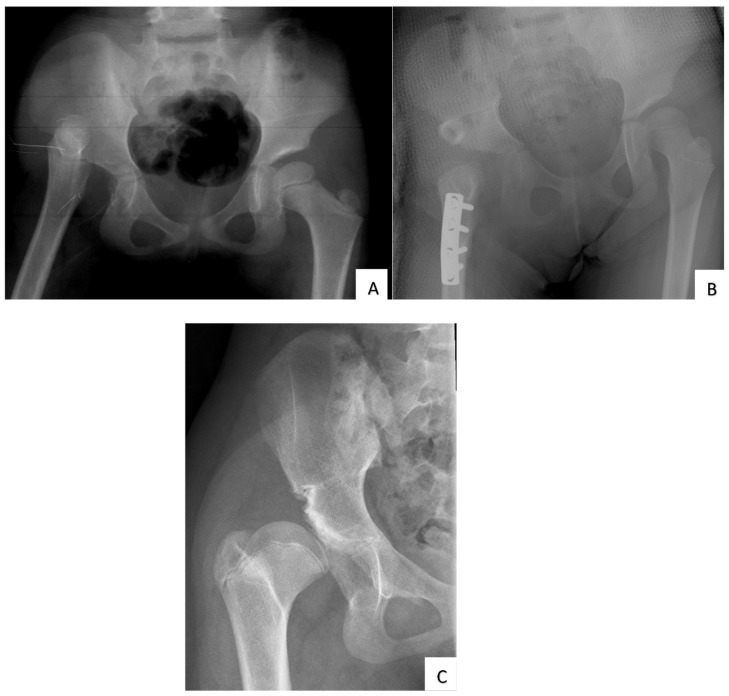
Radiographs of a girl treated with open reduction, femoral shortening, and Salter osteotomy. (A) Pre-operative pelvic radiograph at age 6 years old showing a high right hip dislocation and dysplastic acetabulum. (B) Post-operative radiograph shows the hip is reduced but retroverted. Salter osteotomy with a bone graft taken from the resected femoral shaft. The K-wire fixing the bone graft has been removed. (C) At the age of 13 years, the radiographic outcome is poor (Severin class IV). There are presence of coxa breva, femoral head subluxation, and severe acetabular dysplasia. Her clinical outcome by McKay grading was also poor.
